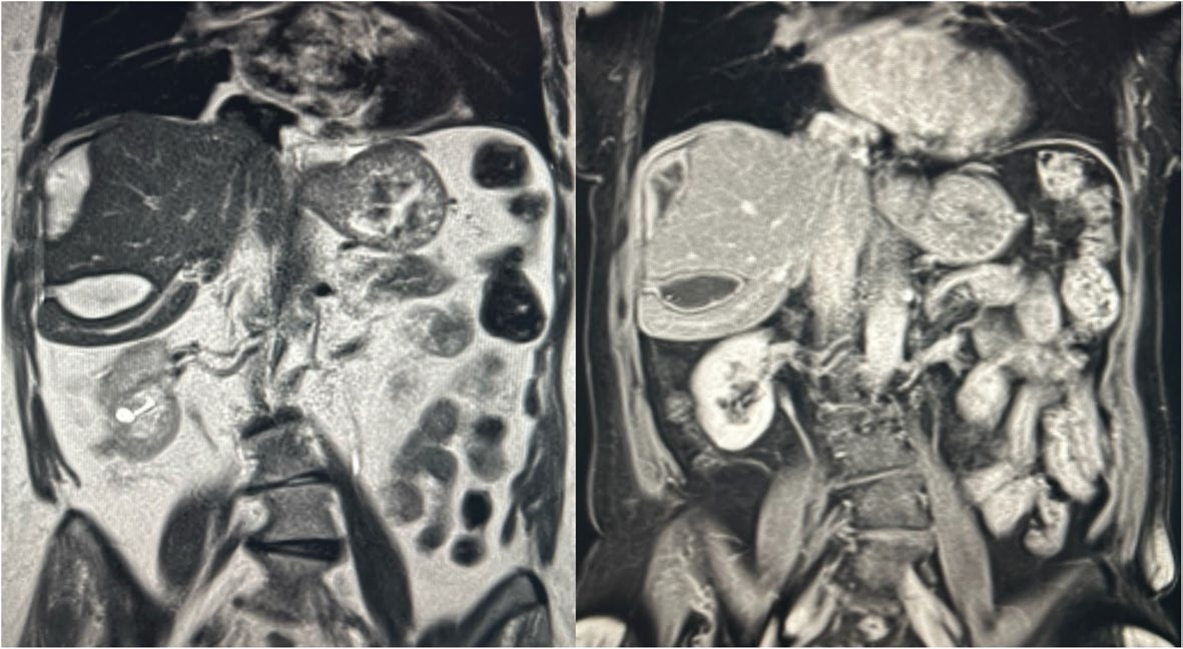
Coronal T2 weighted MRI image (left) and coronal T1 weighted MRI with contrast showing a reduction in haematoma size and no underlying liver lesions
